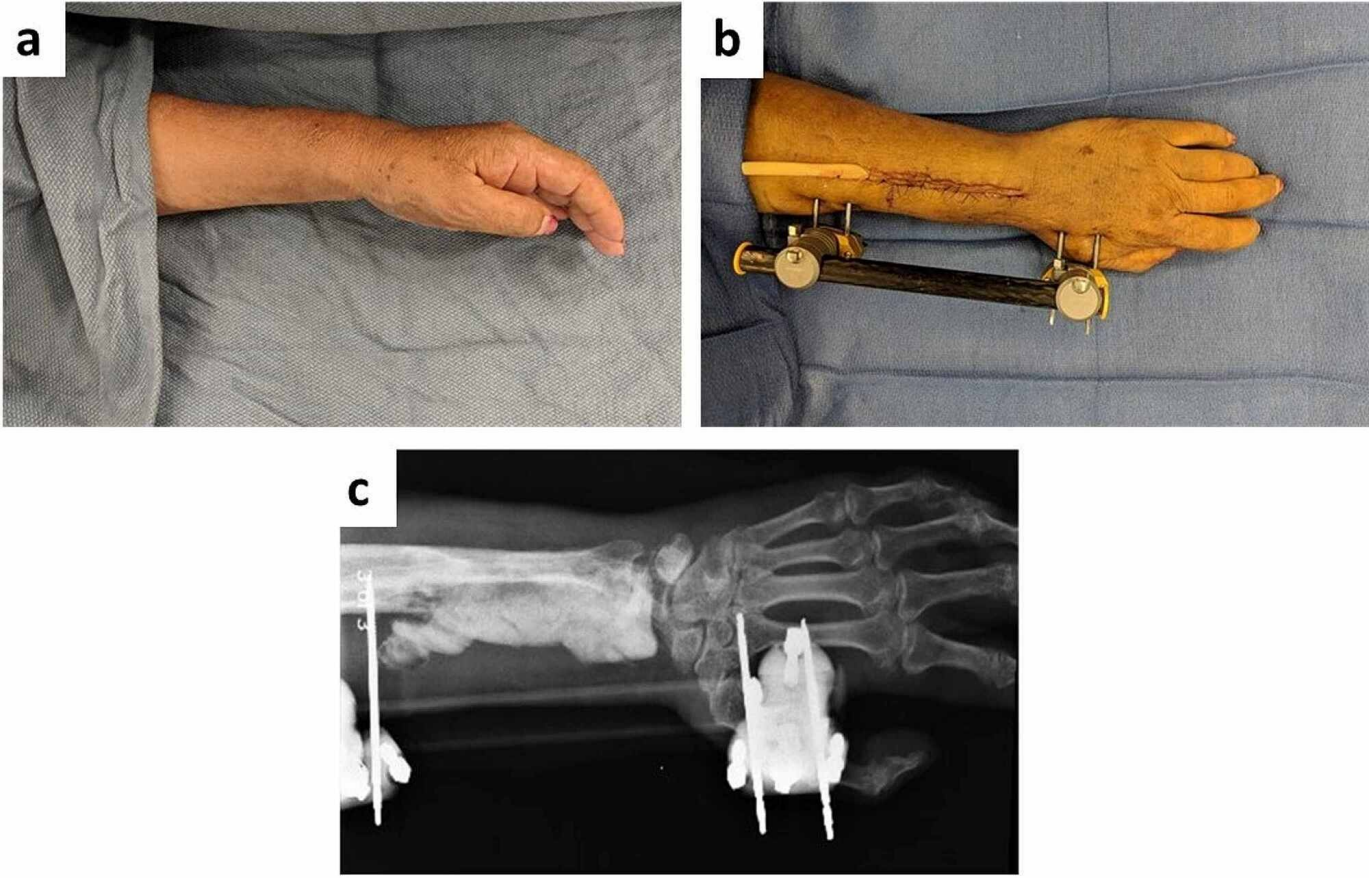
(A) Preoperative image of the left forearm. (B) Postoperative image of the left forearm with external fixator in place after excision of the distal radius, radial shaft, base of lunate, and proximal radius and placement of amphotericin beads. (C) Postoperative X-ray of the left forearm with external fixator in place and placement of amphotericin beads.
